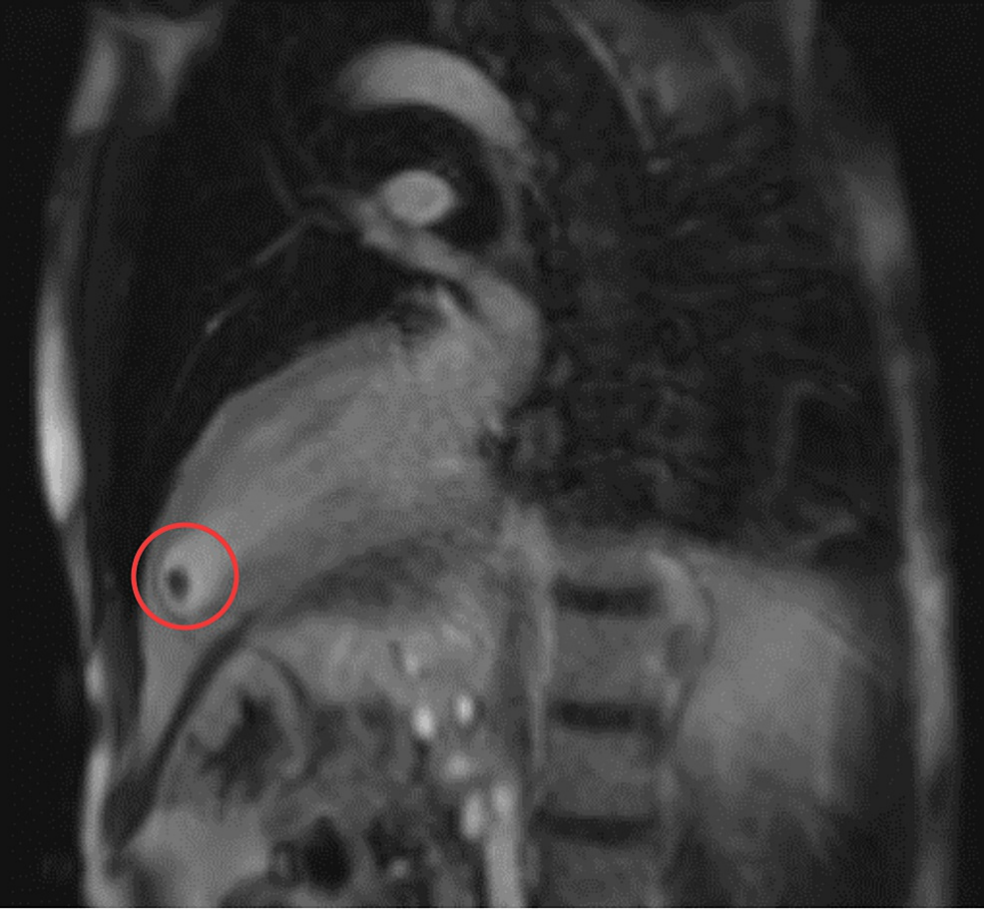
Cardiac MRI showing a small focal outpouching within the left ventricular apex, likely present since a CT exam from 2007. Cardiac MRI before contraction, The non-mobile mass is likely a thrombus. This finding most likely represents a congenital LVD. Within this diverticulum, there is a thrombus. MRI, magnetic resonance imaging; CT, computed tomography; LVD, left ventricular diverticulum (the poor image quality is due to some motion artifact)
